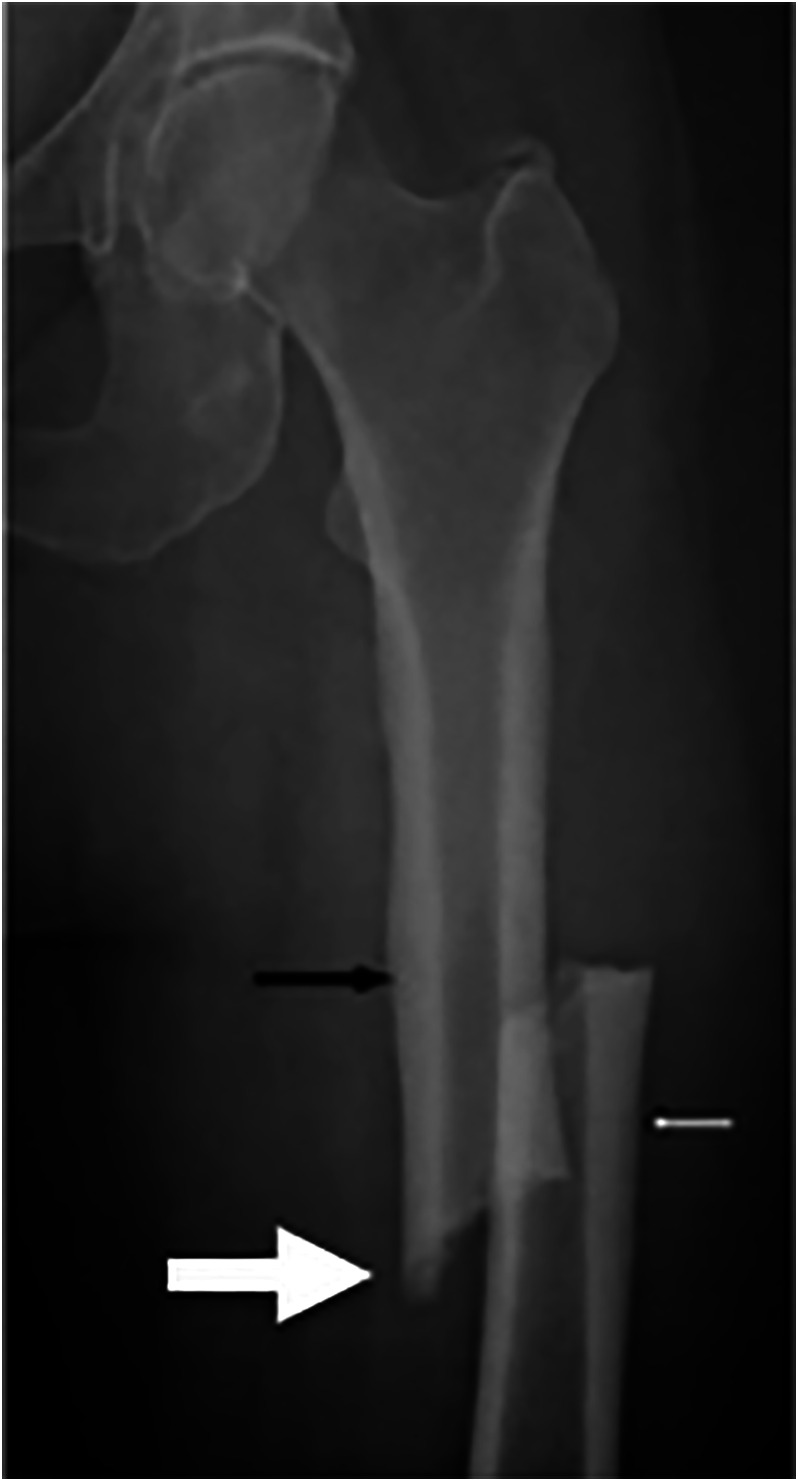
Representative atypical femur fracture radiograph. A 65‐year‐old female patient was taking bisphosphonates for 6 years, with 6 months of progressive prodromal thigh pain. She had seen an orthopedic surgeon 1 month prior to the fracture with pelvic x‐rays showing no evidence of significant osteoarthritis at the time. Note the periosteal thickening of the lateral cortices (black and white arrows), noncomminuted transverse fracture, and the medial cortical spike (big arrow) that are typically seen in atypical femur fractures.^(^
